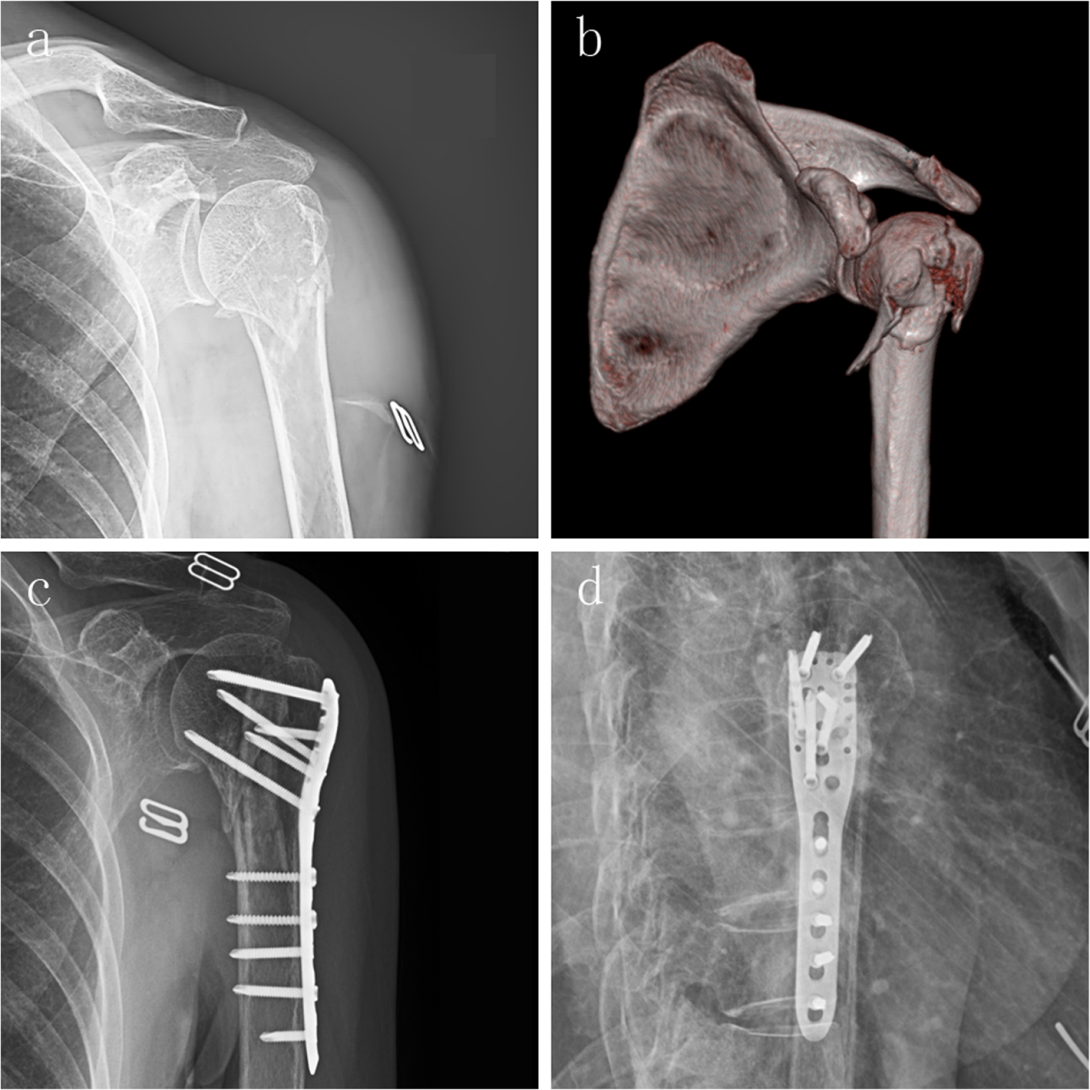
A case of a 3-part fracture with severe metaphyseal comminution. a Radiograph of a displaced 3-part humeral fracture in the left shoulder of a 73-year-old woman. b CT scan, 3-D reconstruction view. (c, d) Radiograph 1 year after surgery
